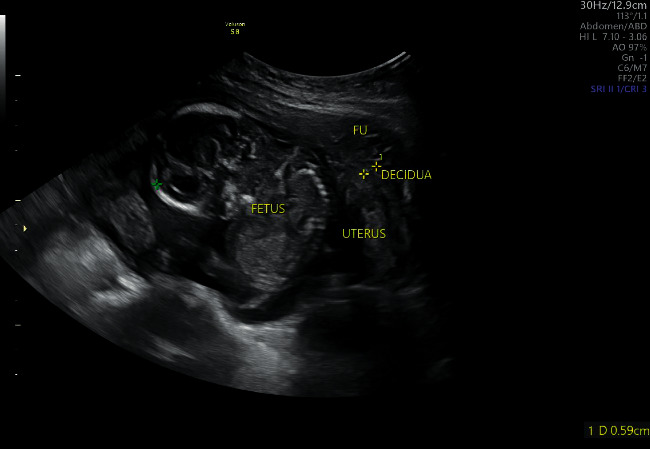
Ultrasonographic image of the abdominal pregnancy.
